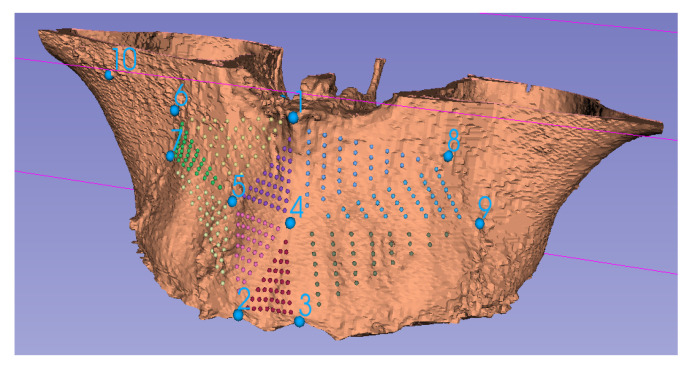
Using CBCT data, the maxillary complex has been rendered in 3D and the semi-landmarks (colored points) have been computed using the ten homologous landmarks (1–10).
